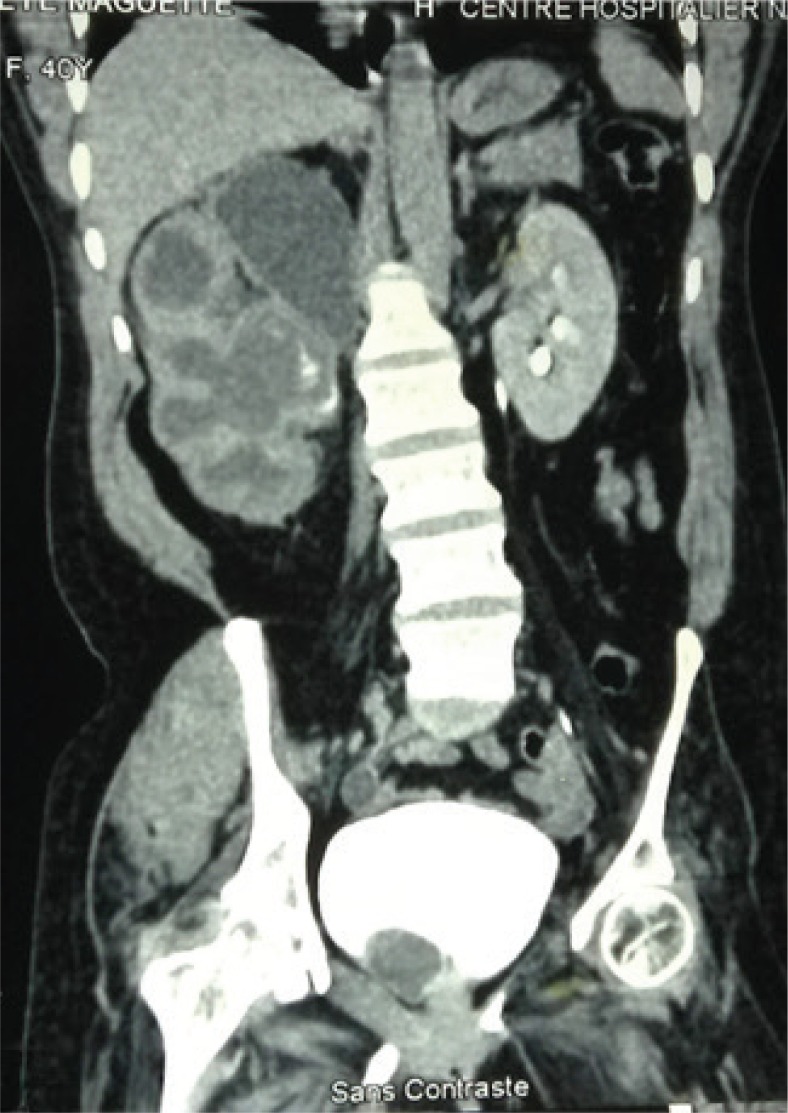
A contrast CT-scan showing a cystic right renal tumor in the upper pole with associated hydronephrosis. Courtesy: Grand Yoff Hospital.
